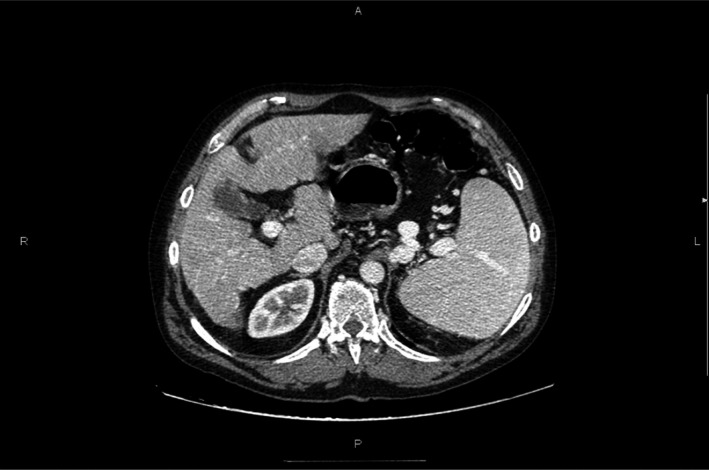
Abdominal CT scan lobular liver, suggesting cirrhosis without nparenchimal, biliary or vascular lesions, normal portal vein and enlarged spleen (19 cm.). Kidney of normal size and morphology. No retroperitoneal or lymphadenopathy and no free peritoneal fluid were observed.
